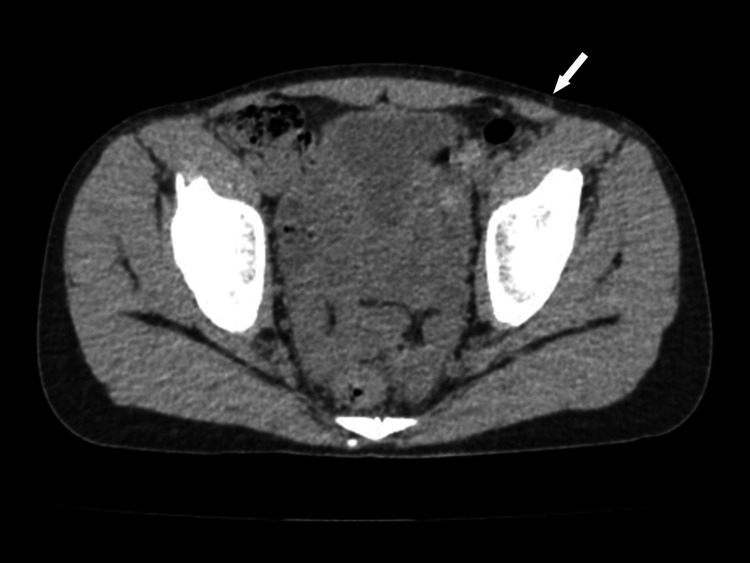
Preoperative CT image in case four showing subtle nerve-like structure at the pain site Axial CT image from case four showing no overt abnormalities in the left rectus abdominis muscle. However, a faint linear structure possibly representing the anterior cutaneous nerve can be seen at the site corresponding to the patient's reported pain (white arrow). Although subtle, this finding supports the clinical suspicion of anterior cutaneous nerve entrapment syndrome.
